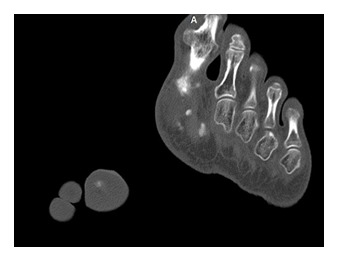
CT aspect. Deformations of the hallucal bone
